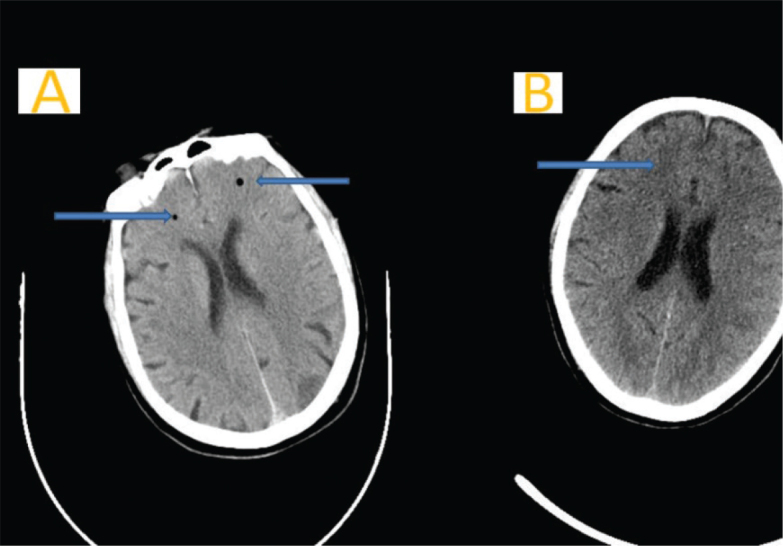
Gas accumulation before and after treatment. (A) Axial non-contrast cranial CT scan obtained on September 19 demonstrates intracranial gas accumulation (arrows); (B) Follow-up CT 24 h post-HBOT reveals complete resolution of intracranial air collections. CT, computed tomography; HBOT, hyperbaric oxygen therapy.
